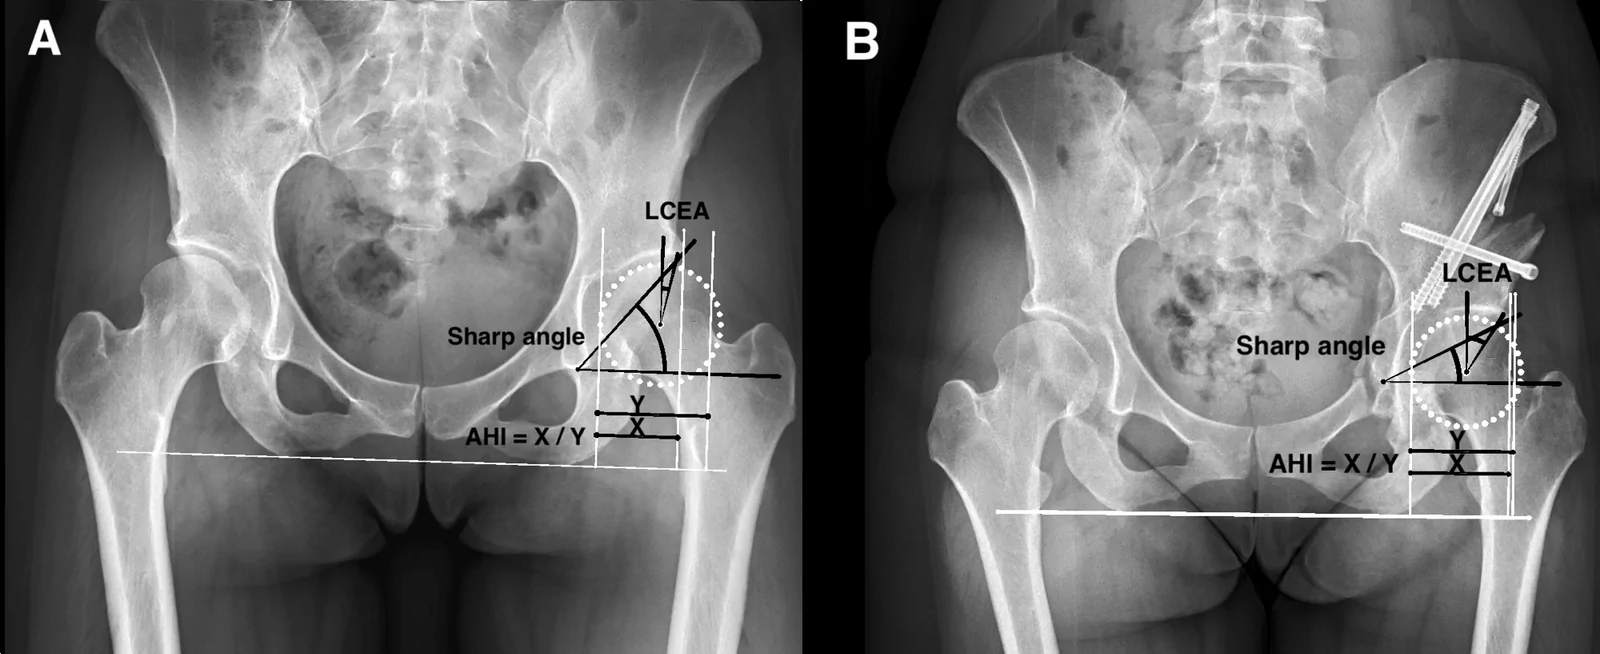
Measurement of the lateral centre-edge angle (LCEA), Sharp’s angle, and acetabular head index (AHI) on a) preoperative and b) Six-month postoperative radiographs from a 33 year-old female. A line connecting the ischial tuberosities was used as the horizontal reference for all measurements. The LCEA is defined as the angle between a vertical line perpendicular to the horizontal reference passing through the centre of the femoral head and a line extending from the femoral head centre to the lateral edge of the sourcil. The Sharp’s angle is defined as the angle between the horizontal reference and a line connecting the inferior aspect of the acetabular teardrop to the lateral edge of the acetabular roof. The AHI is defined as the ratio of the horizontal distance from the medial margin of the femoral head to the lateral edge of the acetabulum to the total horizontal diameter of the femoral head.
